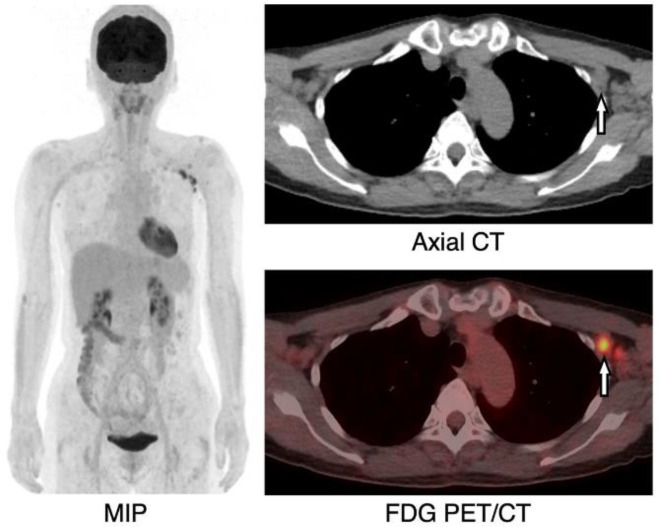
A 55-year-old female, FDG PET/CT (MIP) showed multiple FDG uptakes in eight left axillary lymph nodes from levels 1 to 3 (SUV_max_=2.8–7.9). Axial CT and FDG PET/CT showed the largest of the lymph nodes with the minimum diameter of >10 mm (arrow, short axis: 11 mm, SUV_max_=7.9)
